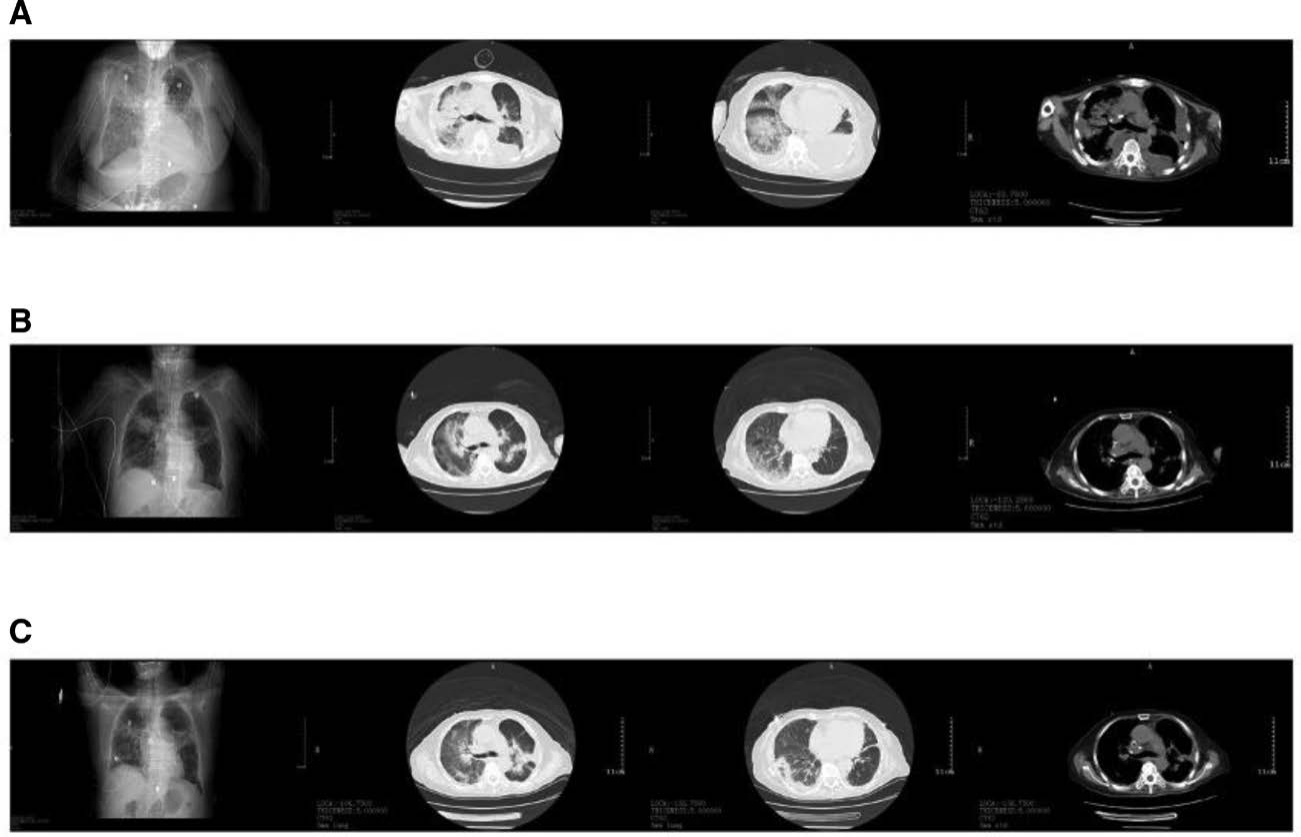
Chest CT during hospitalization (A) Chest CT on the 3rd day after admission (B) Chest CT on the 10th day after admission (C) Chest CT on the 20th day after admission. CT = computed tomography.
